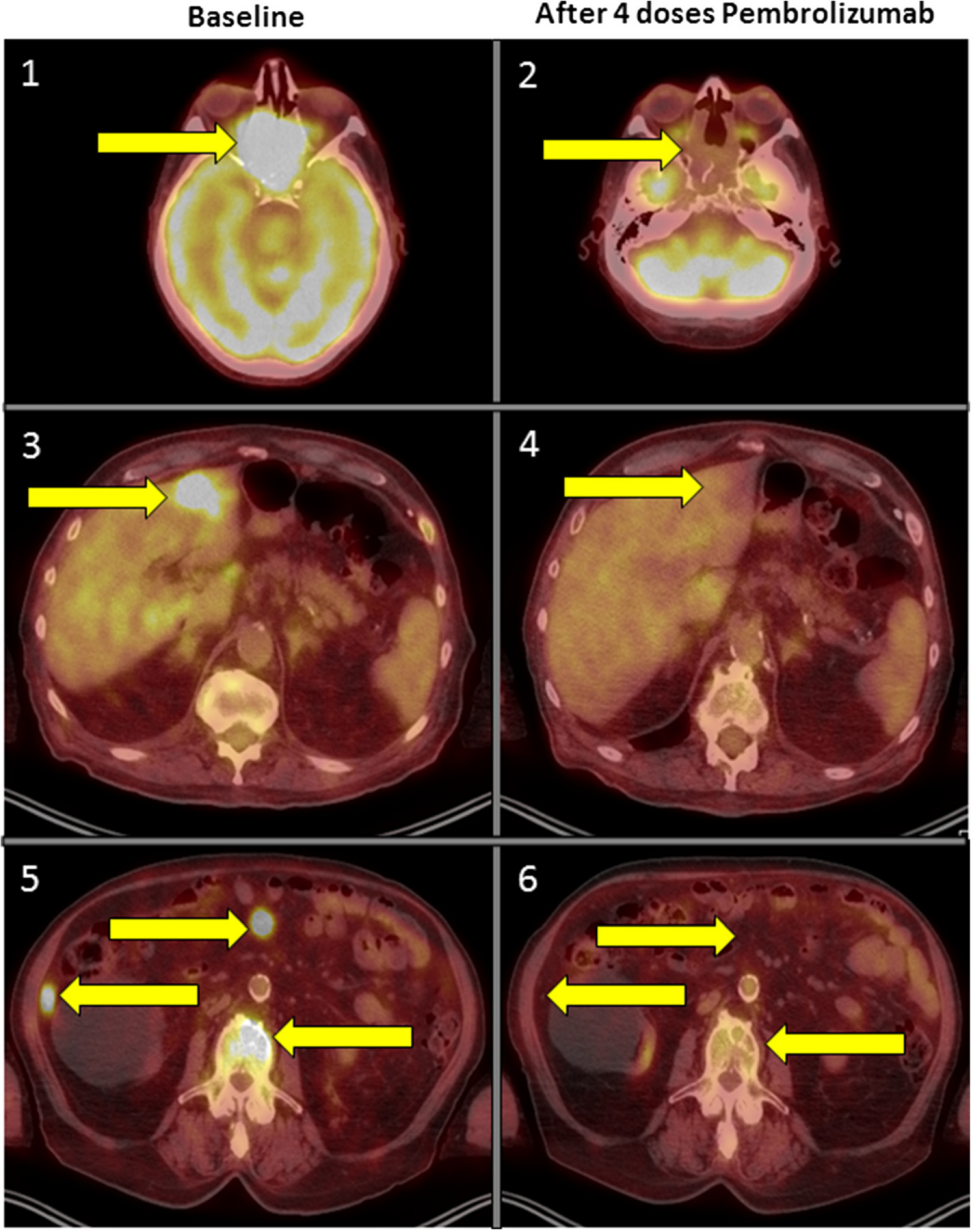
Baseline (left column) and post-pembrolizumab (right column) therapy representative PET/CT images. Side-by-side comparative images of PET/CT scan at baseline and after the first four doses of pembrolizumab. Yellow arrows denote sites of active or previously identified melanoma deposits. (1 and 2) Primary sphenoethmoidal mass showing a CR after treatment. (3 and 4) CR in a left hepatic lobe liver metastasis. (5 and 6) CR in two intra-abdominal metastatic implants and in a vertebral metastasis
